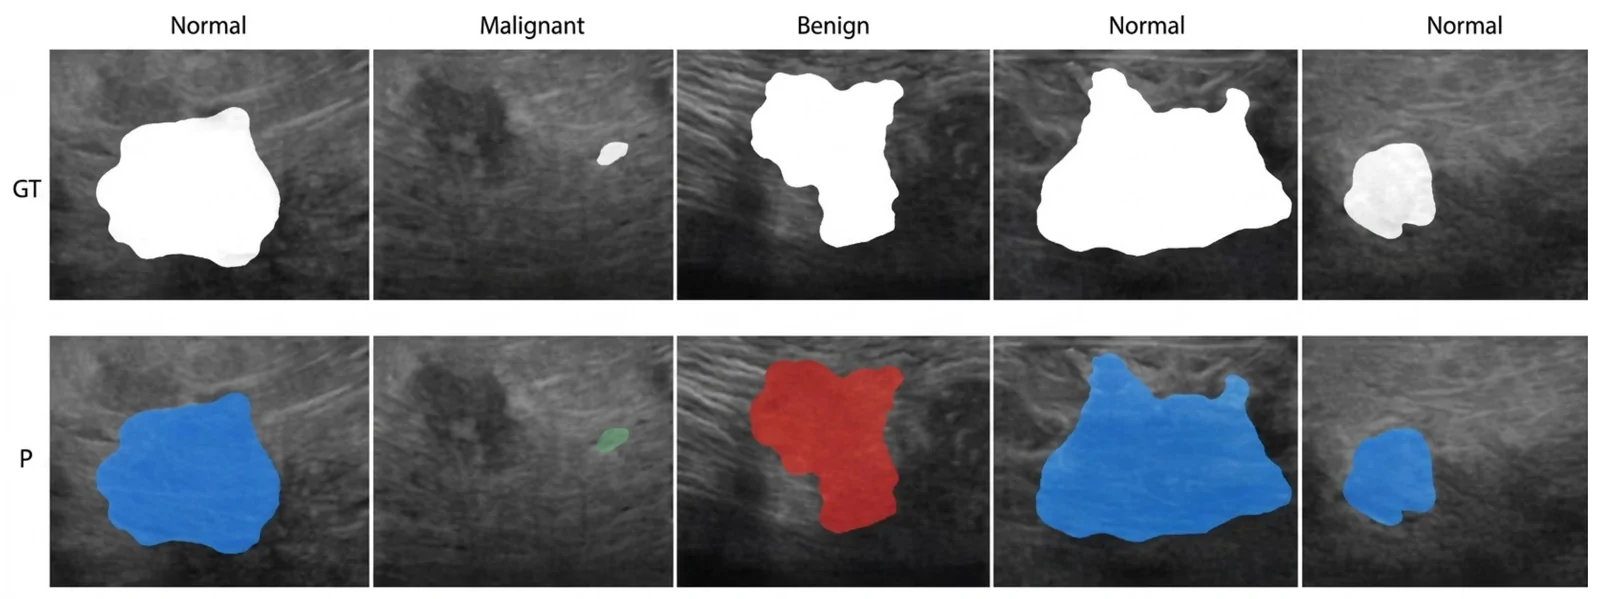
Predictions. Each row: input ultrasound image with ground-truth mask (white) and predicted mask, with predicted class (BENIGN in red/MALIGNANT in green/NORMAL in blue).
